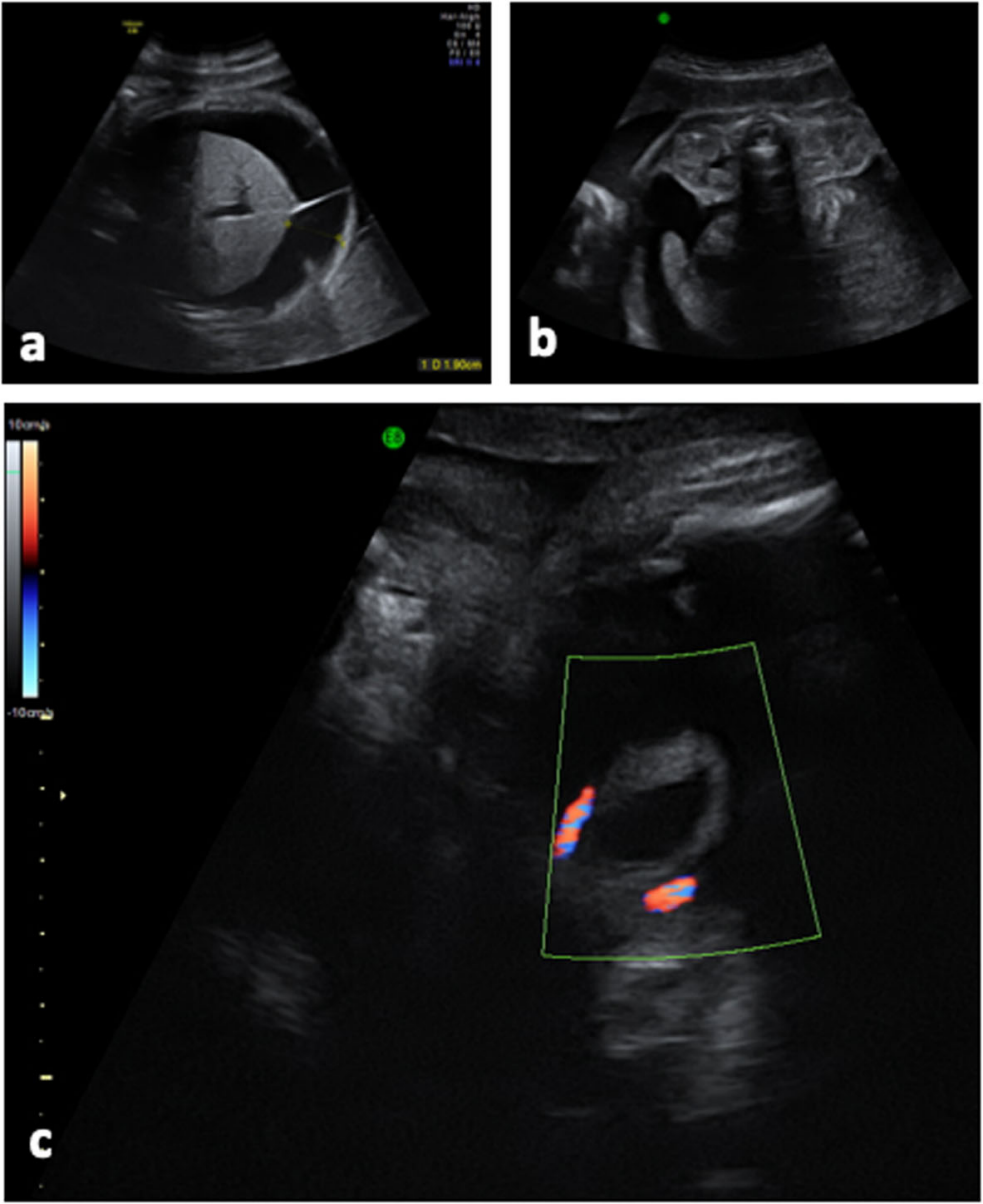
Fetal ultrasound at 30 weeks and 1 day. (a) Fetal ascites, (b) fetal ascites, normal appearance of the kidneys, (c) small capacity bladder with thickened walls
